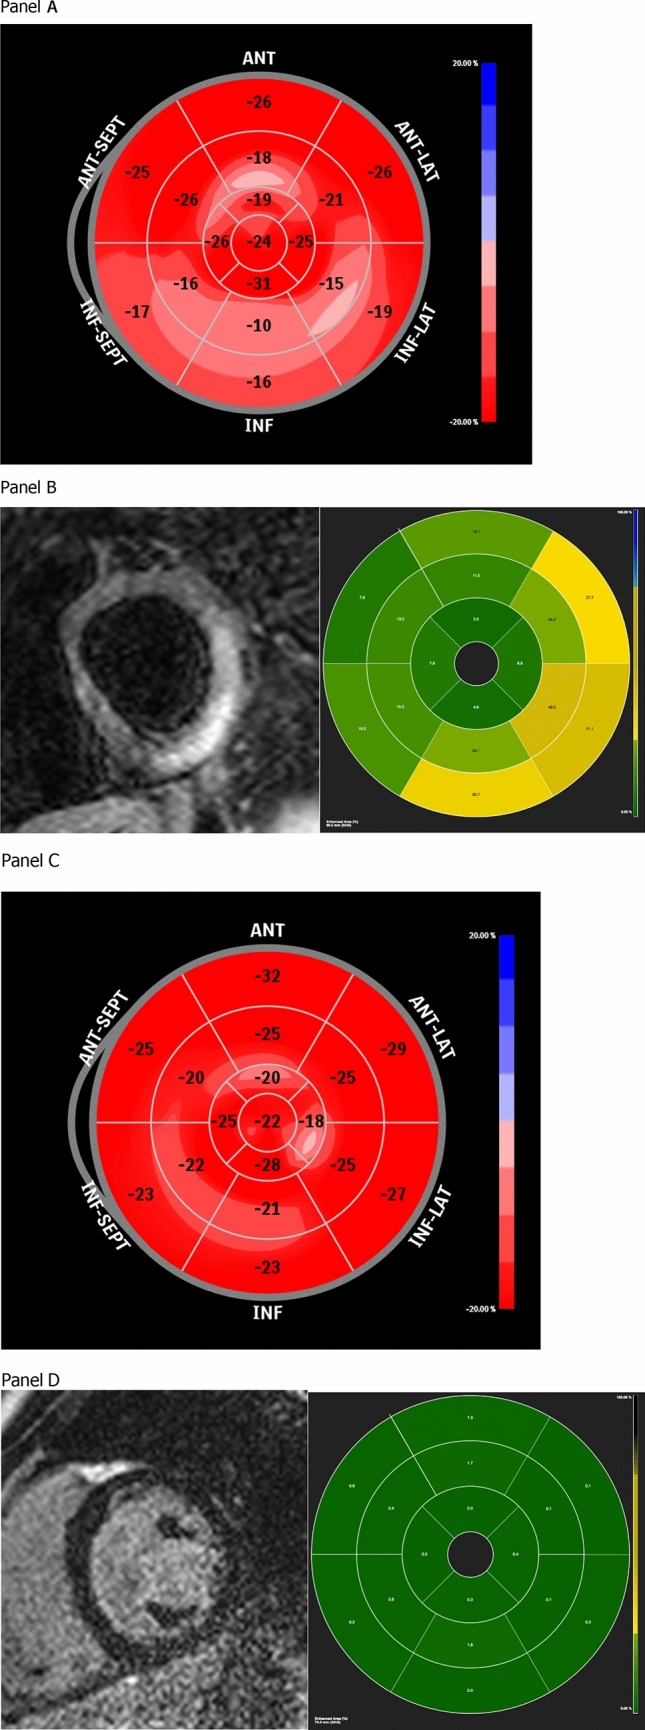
Example of patient with recovered systolic dysfunction at follow-up (Panel A and C images acquired with QLab Cardiac Analysis ver.10, Philips Heathcare Inc., Panel B and D images acquired with CMR42, Circle Cardiovascular Imaging, Calgary, AB, Canada). (A) Evidence of impaired GLS at baseline echocardiographic evaluation. (B) Evidence of significant focal edema at baseline CMR (qualitative and quantitative). (C) Evidence of recovered GLS at follow-up echocardiographic evaluation. (D) Evidence of no focal fibrosis at follow-up CMR (qualitative and quantitative).
